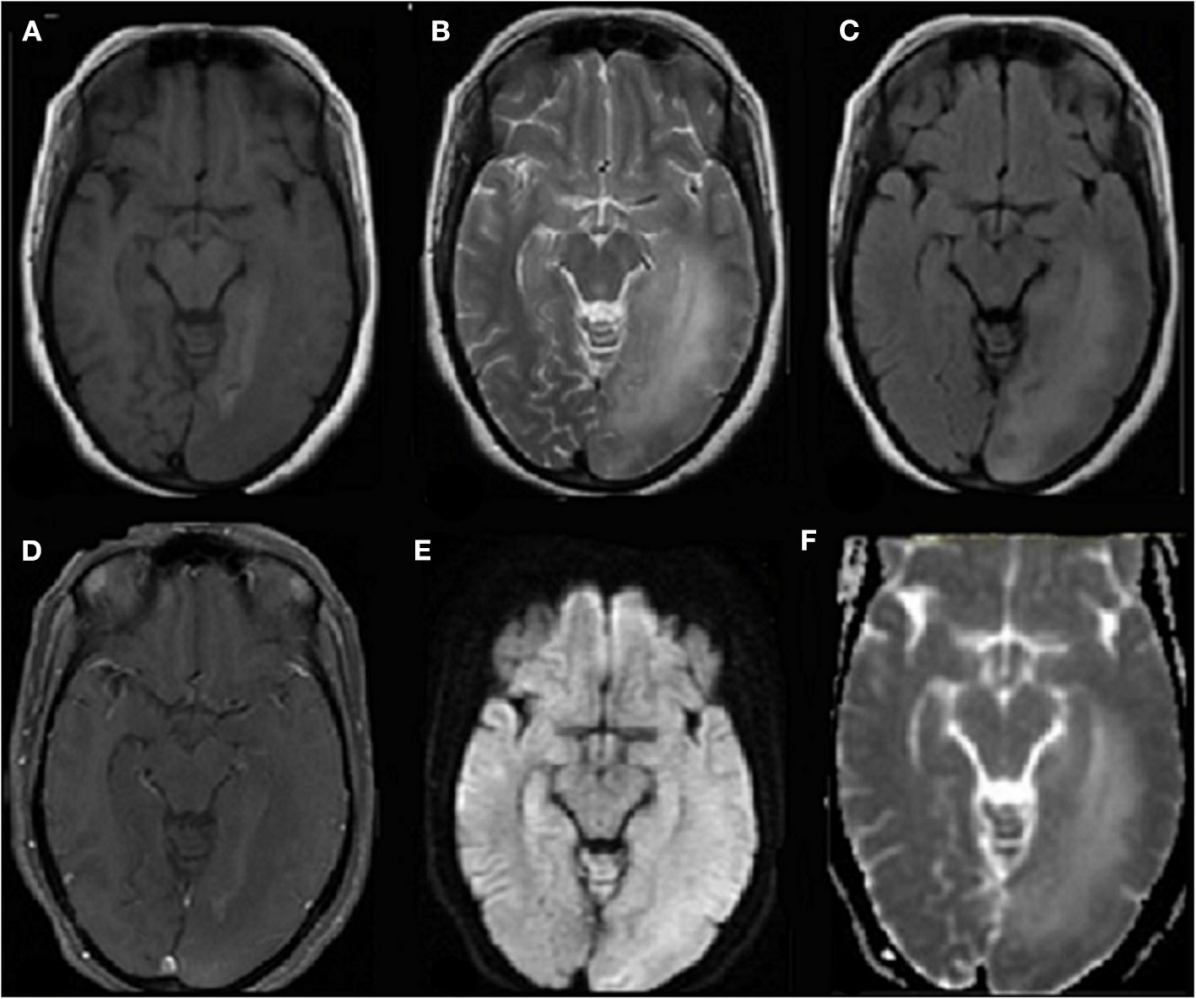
Axial T1 weighted (A) MR image shows hypointensity in left parieto-occipital region in subcortical and deep white matter with overlying sulcal effacement and corresponding hyperintensity on T2 weighted image (B) and FLAIR (C) images. No associated contrast enhancement seen on contrast-enhanced T1-weighted MR image (D) with no restricted diffusion on diffusion-weighted MR image (E), and apparent diffusion coefficient (ADC) map (F).
